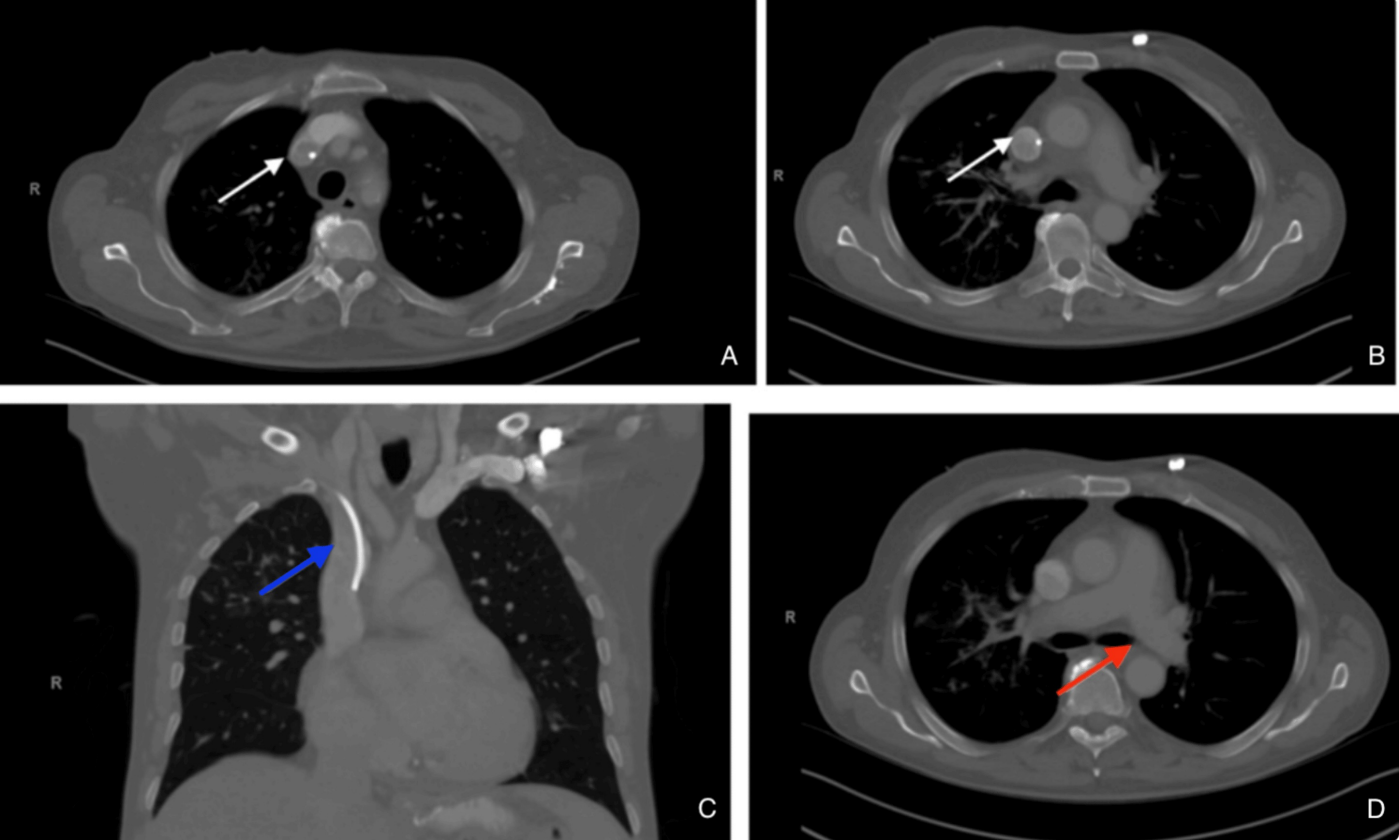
CT chest demonstrating appropriate placement of the catheter A and B: catheter within the right subclavian vein (marked by a white arrow); C: intact catheter terminating in the correct location near the cavoatrial junction (marked by a blue arrow); D: no evidence of a fractured piece of catheter within the left pulmonary artery (marked by a red arrow).
